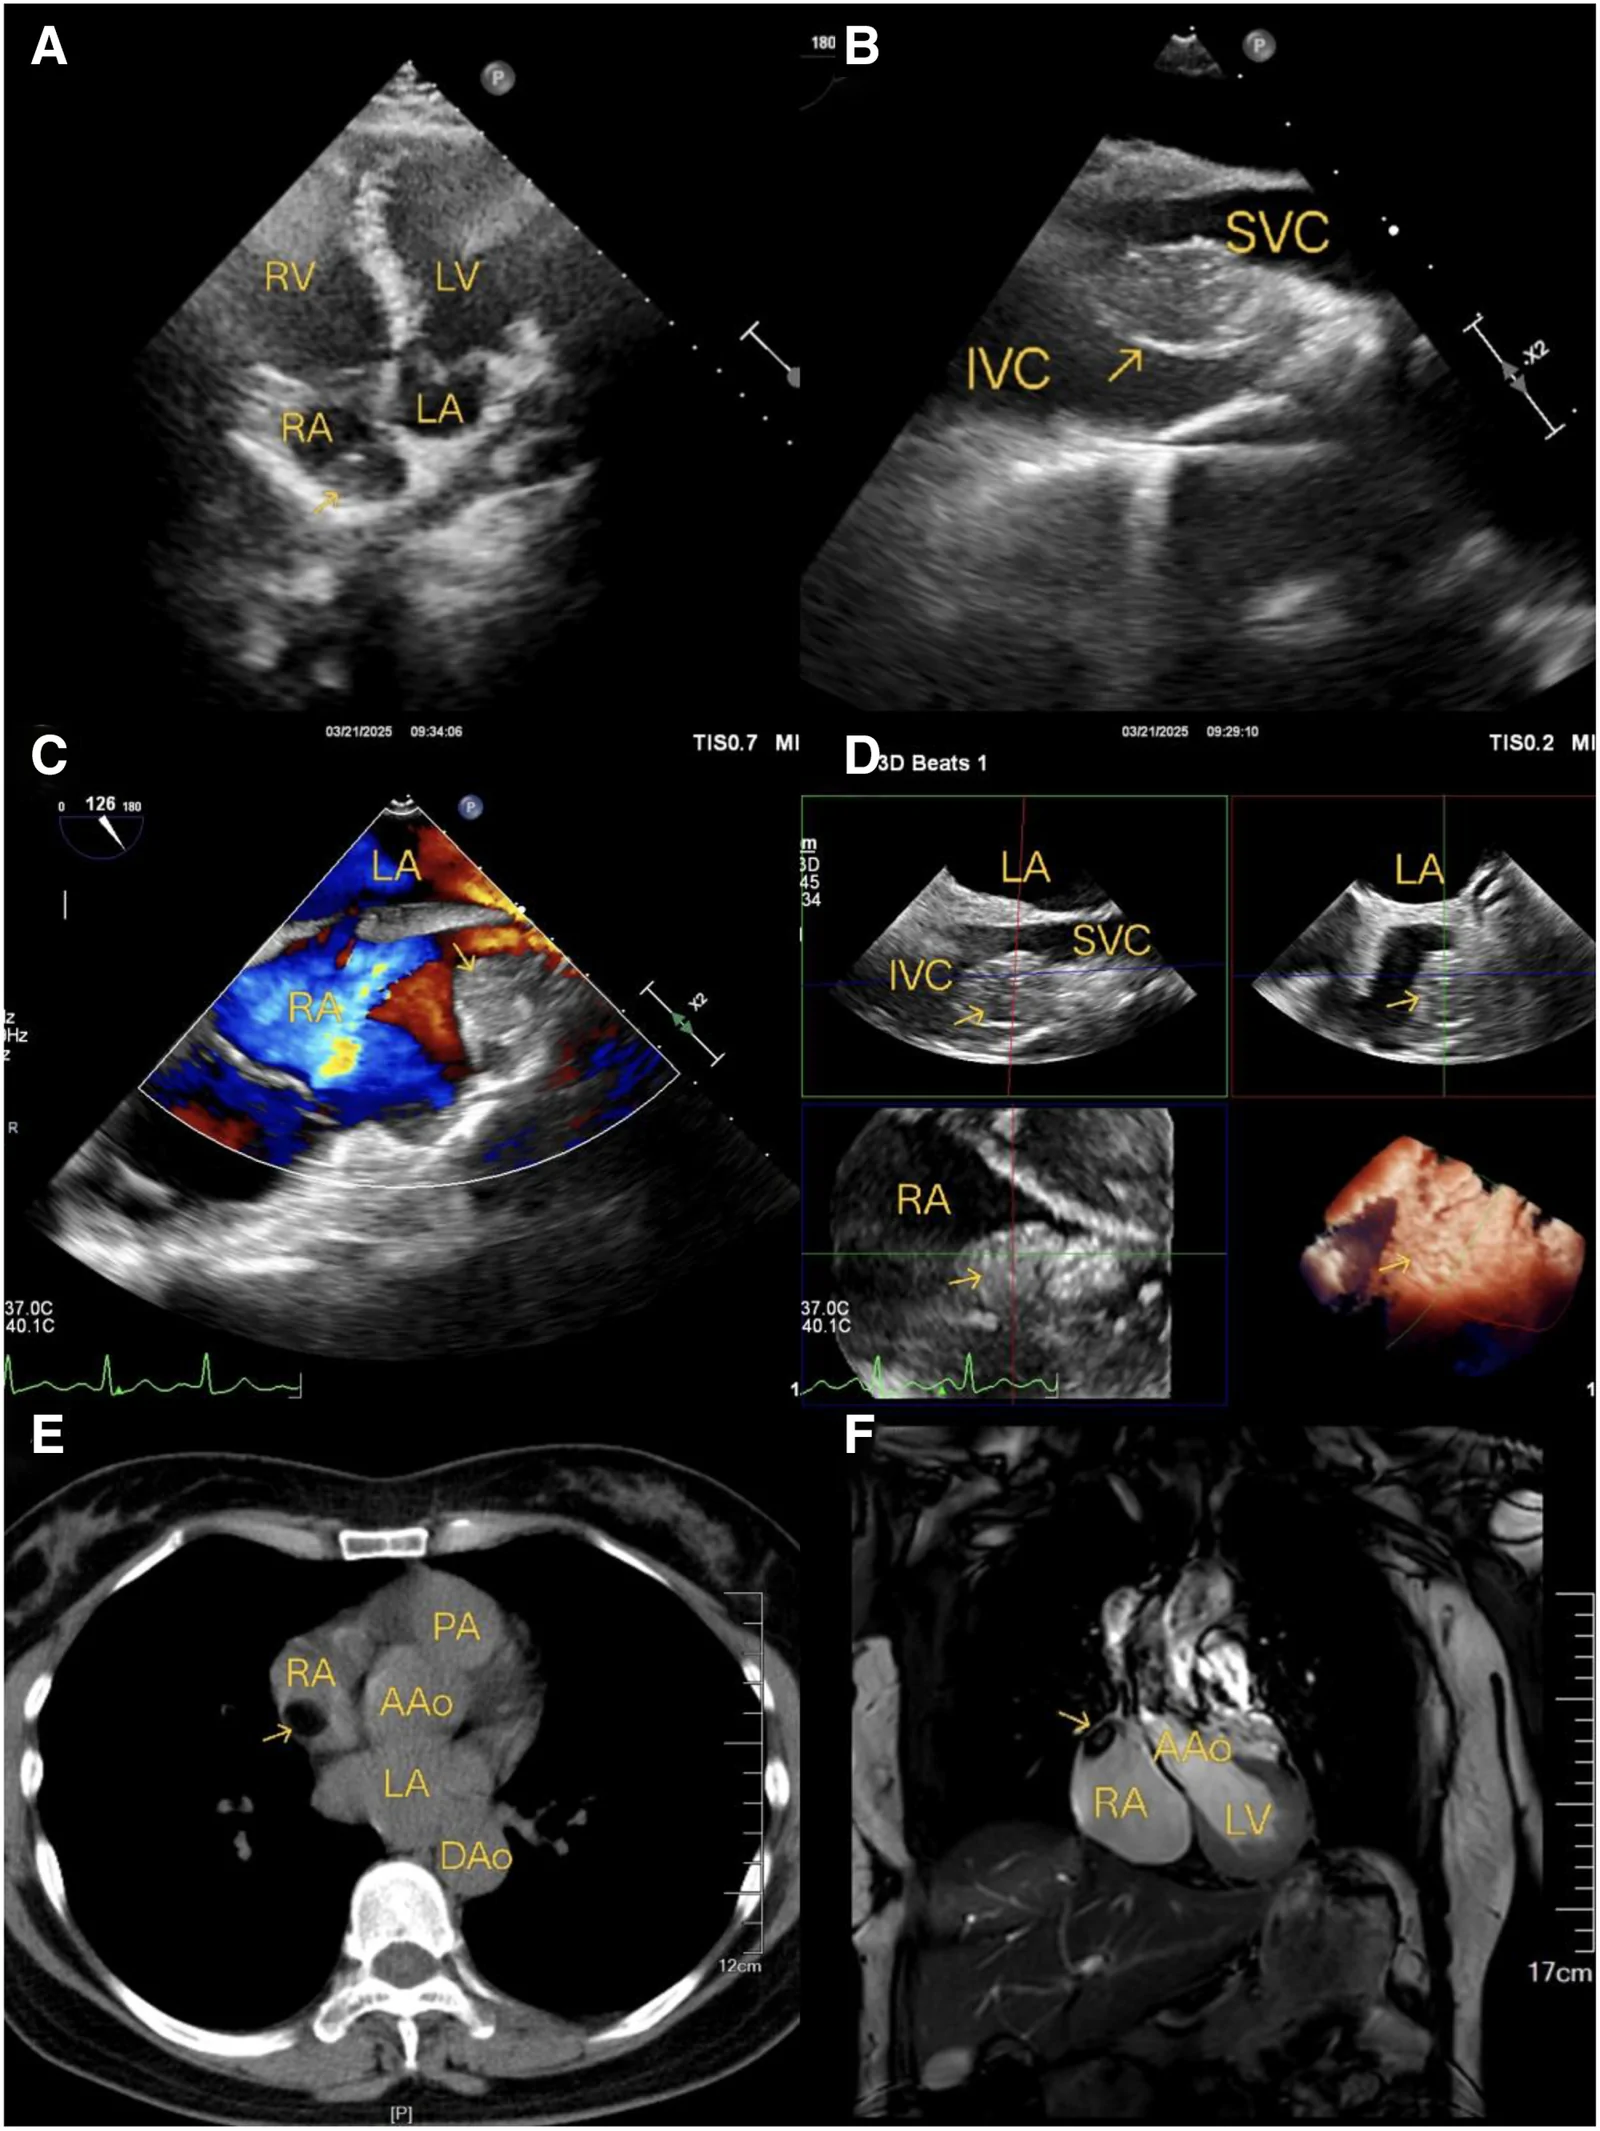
(A) Apical 4-chamber view on transthoracic echocardiography (TTE) showing a hypoechoic to isoechoic mass within the right atrium, adjacent to the orifice of the superior vena cava. (B-D) Two-dimensional and 3-dimensional transesophageal echocardiography (TEE) demonstrating a hypoechoic to isoechoic mass with well-defined borders at the superior vena cava-right atrial junction. The lesion appears pedunculated and shows no significant internal flow signal on Doppler imaging. (E) Mediastinal window on computed tomography (CT) revealing a relatively well-defined, fat-attenuation nodule located in the right atrium. (F) Cardiac magnetic resonance (CMR) imaging on a T2-weighted fat-suppressed sequence showing low signal intensity of the mass. The arrow indicates the location of the mass. AAo = ascending aorta; DAo = descending aorta; CMR = Cardiac magnetic resonance; CT = Computed tomography; IVC = inferior vena cava. LA = left atrium; LV = left ventricle; RA = right atrium; RV = right ventricle; SVC = superior vena cava; TTE = Transthoracic echocardiography.
